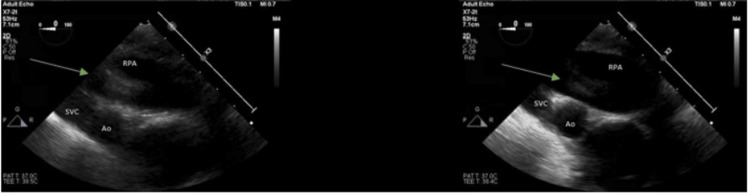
Upper esophageal ascending aorta view. A transesophageal echocardiogram was performed intraoperatively status post cardiac arrest, revealing biventricular systolic dysfunction, severe septal wall hypokinesis, 3+ mitral and tricuspid regurgitation, dilated left and right atria, and left ventricle ejection fraction of 30 ± 5%. A suspected clot in the right pulmonary artery (green arrow).
